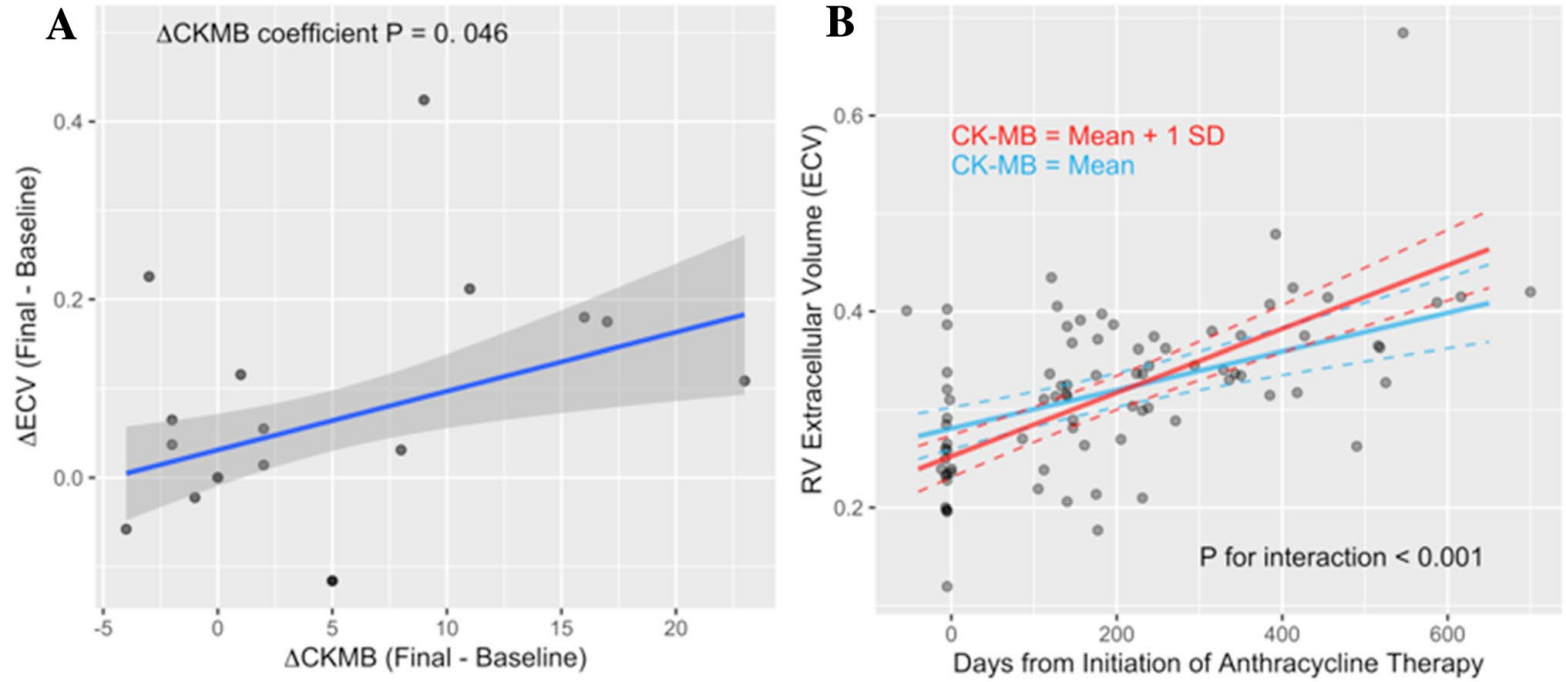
Changes of RV ECV and their association with CK-MB. (A): the change of ECV in the RV between the baseline MRI study and the final CMR (ΔECV) in each patient correlated with the change of CK-MB (ΔCK-MB) a serum biomarker of myocardial injury. (B) RV ECV increased approximately linearly with time from anthracycline therapy. The rate of RV ECV expansion over time after anthracycline therapy (*P* < 0.001 for follow-up-time coefficient estimate) increased in proportion to the serum level of CK-MB activity (*P* < 0.001 for interaction of follow-up time with log-transformed CK-MB level). The longitudinal change of RV ECV was modeled with a linear mixed effects model that included follow-up time (days), log-transformed CK-MB and an interaction term as predictors. The blue and red lines show the predictions from this model for two levels of CK-MB corresponding to the overall mean (blue) of CK-MB and a one standard deviation higher level (red), respectively.
